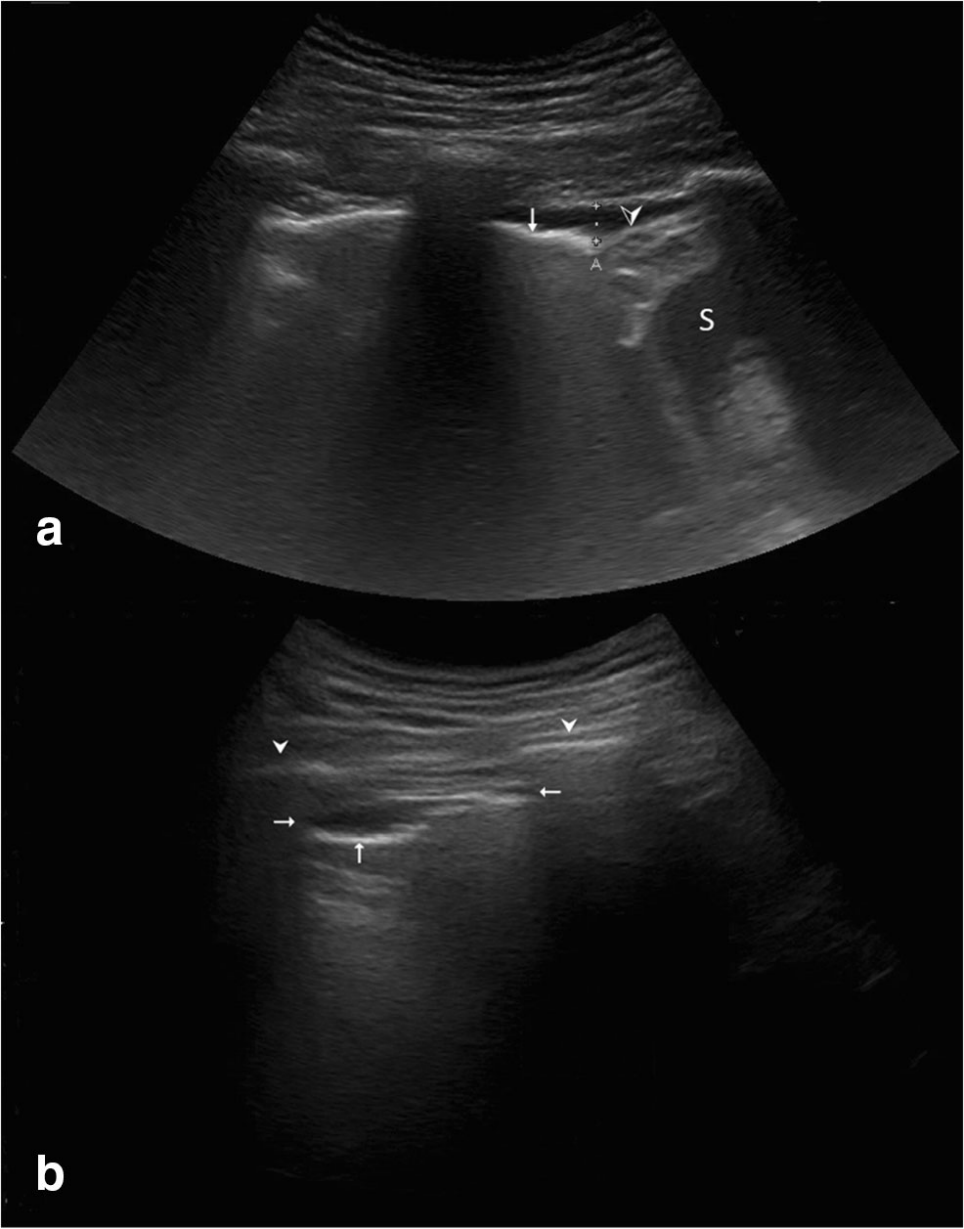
Pleural pathologies observed. Posterior lung scans in the sitting patient. (a) Pleural effusion in the costodiaphragmatic recess in the upright patient, posterior longitudinal view. Distance “A” (dotted line) displays the largest sagittal extent (between chest wall and diaphragm) of this small effusion, which measures 6 mm. Vertical arrow: Visceral pleural line. Arrowhead: Diaphragm. S: Spleen. Note that this effusion was too small to be seen on CT in the supine patient; as fluid collects in the costodiaphragmatic recess in the sitting patient, it was detected using LUS. (b) Pleural plaque in posterior lung scan. Two anechoic lesions (horizontal arrows) are seen adjacent to the parietal pleura. The differential diagnosis of consolidation is dismissed with dynamic visualization of the visceral pleura (vertical arrow) sliding past the stationary lesion; the differential diagnosis of an effusion is dismissed due to the stationary nature of the lesion, (1) not descending into the costodiaphragmatic recess upon inspiration and (2) lack of respiration-dependent expansion and contraction
